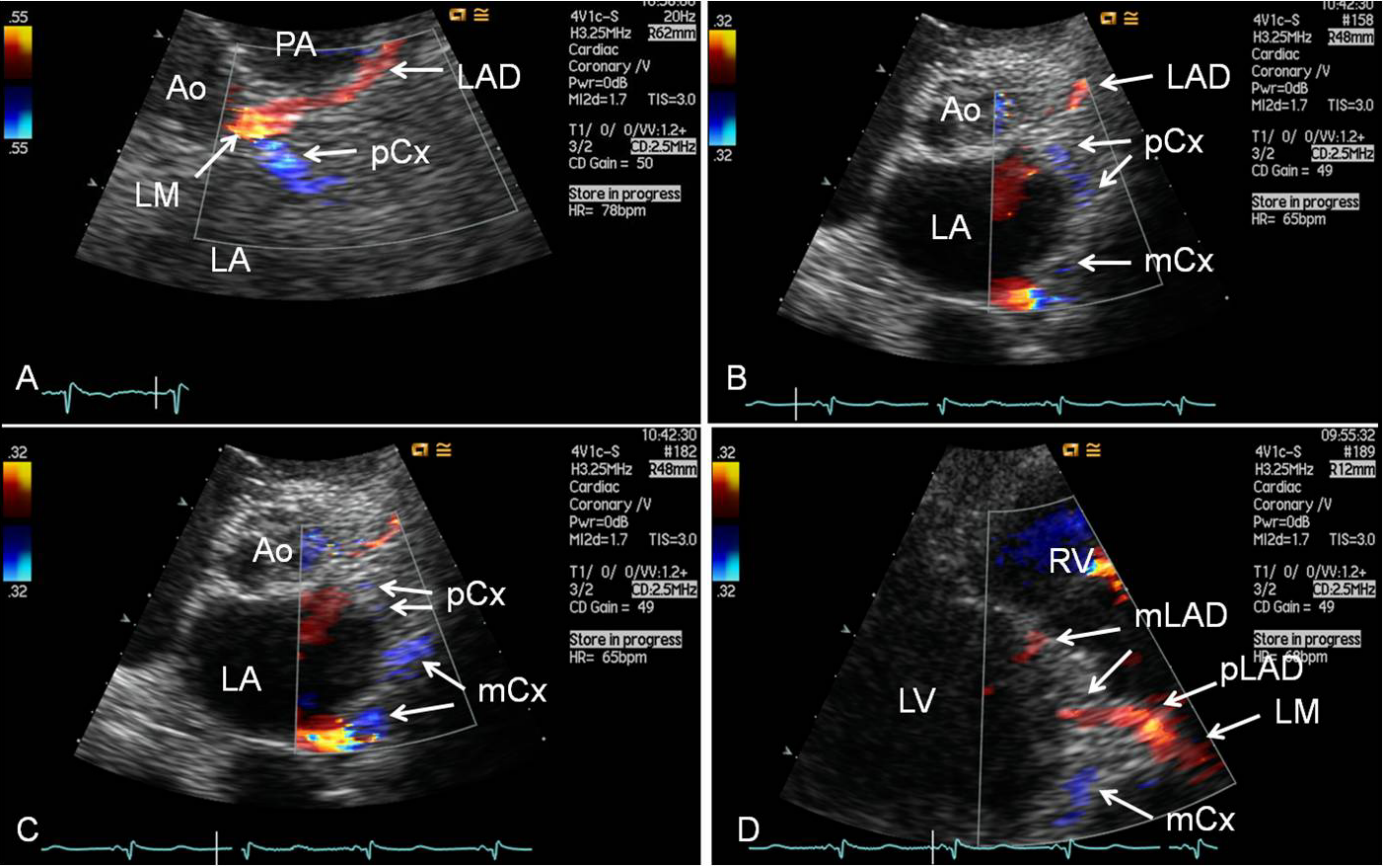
Examples of antegrade coronary artery flow in the proximal and middle segments of Cx. (A) In modified parasternal short-axis view focusing on the area adjacent to the left sinus of Valsalva the proximal circumflex coronary artery (pCx) is seen leaving the left main coronary artery (LM). (B and C) In modified parasternal short-axis views the proximal and middle segments of the circumflex coronary artery (Cx) are found passing caudally in the lateral atrioventricular sulcus. (D) From parasternal modified long-axis view focusing on the lateral atrioventricular sulcus the middle Cx (mCx) is seen traversing caudally. Ao = aortic root/valve; LA = left atrium; LAD = left anterior descending coronary artery; LV = left ventricle; mLAD = middle segment of LAD; PA = pulmonary artery; pLAD = proximal segment of LAD; RV = right ventricle.
